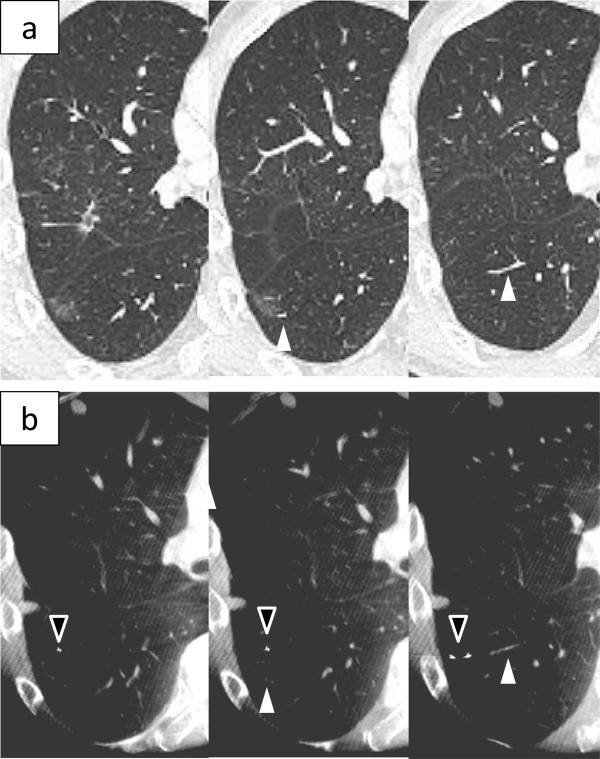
A GGN without a solid part. Preoperative CT shows a GGO lesion, 10 mm in diameter, without a solid part (a). The O-arm could not show the lesion. However, the location of the tumor could be inferred by comparing the positions of the peripheral pulmonary vessels (white arrowhead) or the bronchus near the tumor on the preoperative and intraoperative CT images (b). A PDS needle is placed as a marker (black arrowhead).
